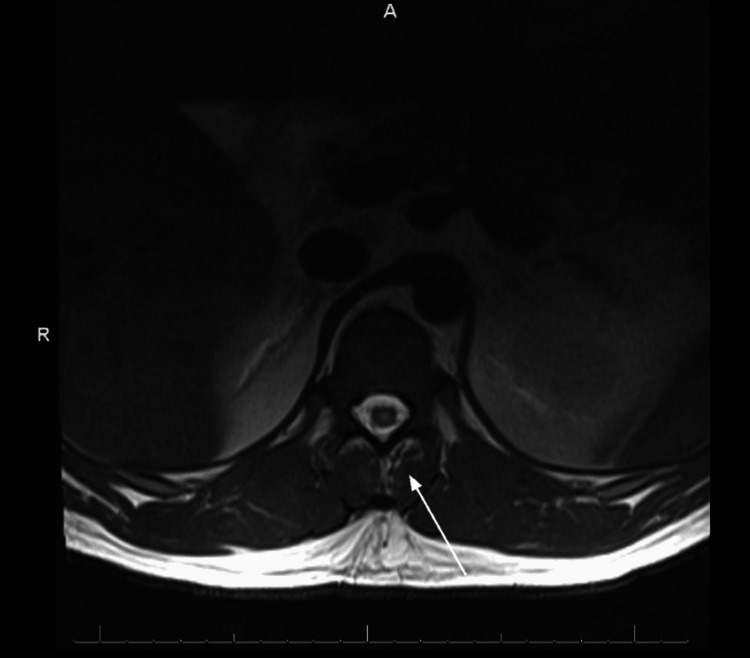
Lumbar spine MRI showing enhancement of the posterior paraspinal muscles on the left side at the L4-L5 level. No osseous enhancement or abscess was present.
